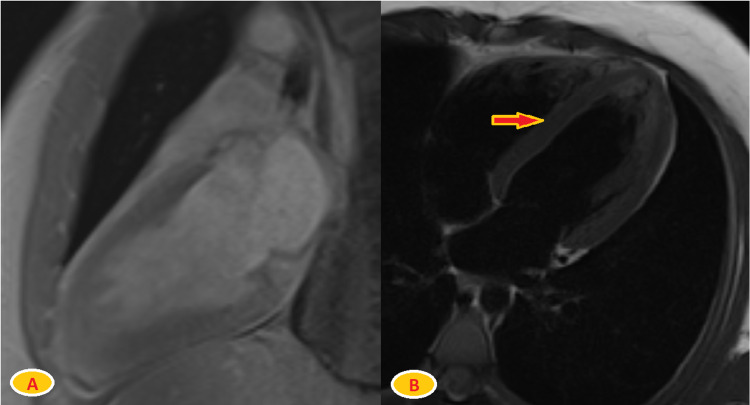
Cardiac MRI using bright blood, dark blood, T1 mapping, and delayed post-contrast signaling A) Long access post-contrast enhancement view with no Gadolinium enhancement. B) T2-weighted image with no abnormal signals in the myocardium (arrow).
